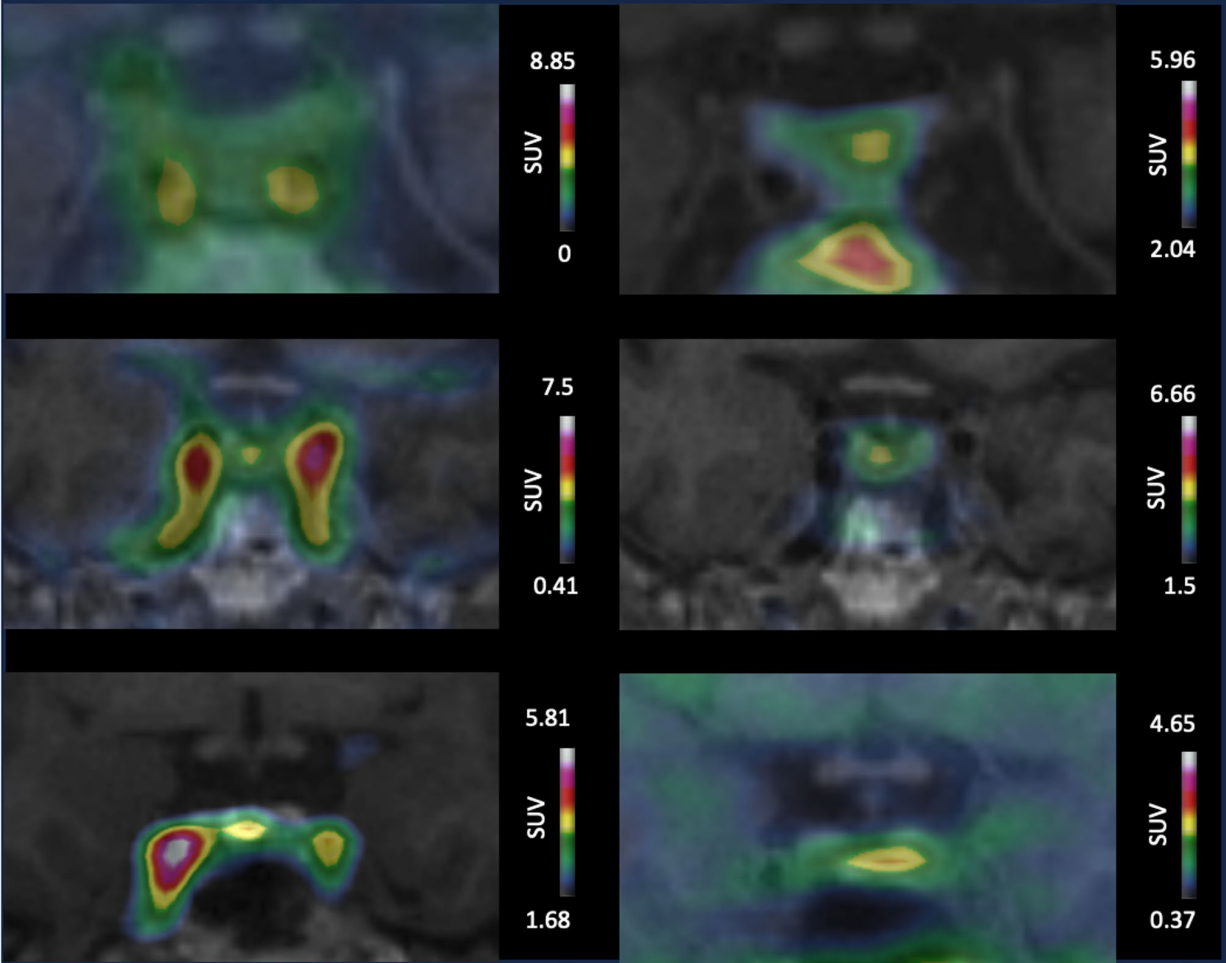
Early and late superimposed [^11^C] MET PET/MRI images for PitNET localization. First column shows early coronal [^11^C]MET PET/MRI images. Second column displays corresponding late [^11^C]MET PET/MRI images. First row: Patient 11 presents no focal uptake on early image but left-sided focal uptake on late image confirmed by pathology. Second row: Patient 10 presents clear right focal uptake, concordant on early and late images confirmed by pathology. Third row: Patient 7 presents clear right-sided focal uptake on early image confirmed by pathology, which was discordant from a left focal uptake on late images. MET: Methionine, SUV: standardized uptake values, PitNET: pituitary neuroendocrine tumors.
